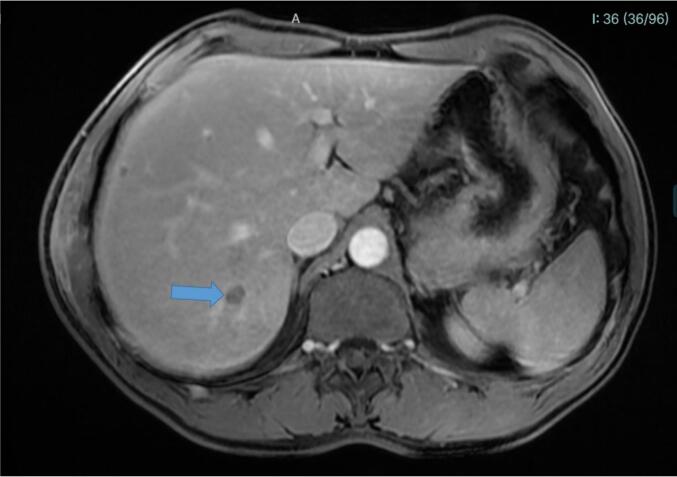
Gadolinium-injected Liver Abdominal Magnetic Resonance Imaging (tardive Lava-Flex T1 sequence) in a patient diagnosed with fasciolosis. The figure is notable for the presence of a non-enhancing lesion consistent with parenchymal abscess (arrow).
